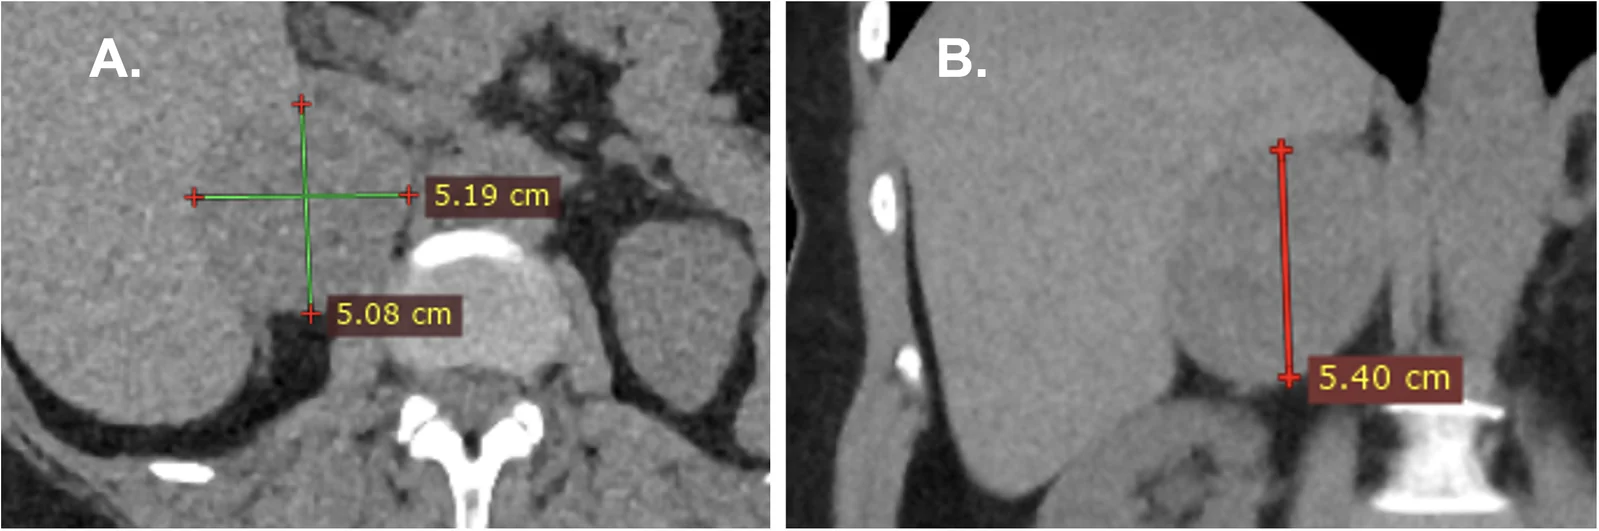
Preoperative computed tomography (CT). Axial (A) and coronal (B) CT images demonstrating a heterogeneous 5 cm mass in the right adrenal gland, consistent with a pheochromocytoma, prior to the onset of the adrenergic crisis.
